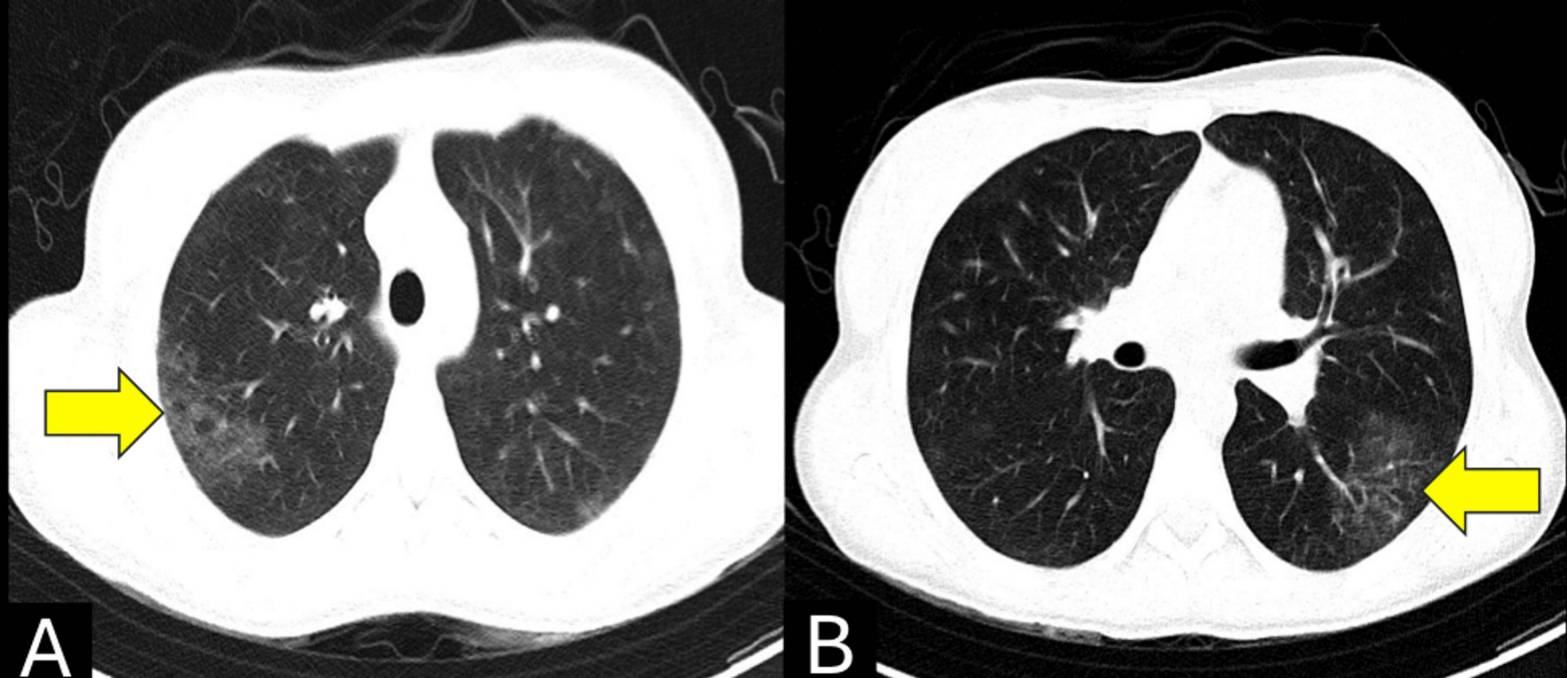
Chest CT demonstrates persistent bilateral ground-glass opacities (yellow arrows), seen seven weeks after initial presentation
